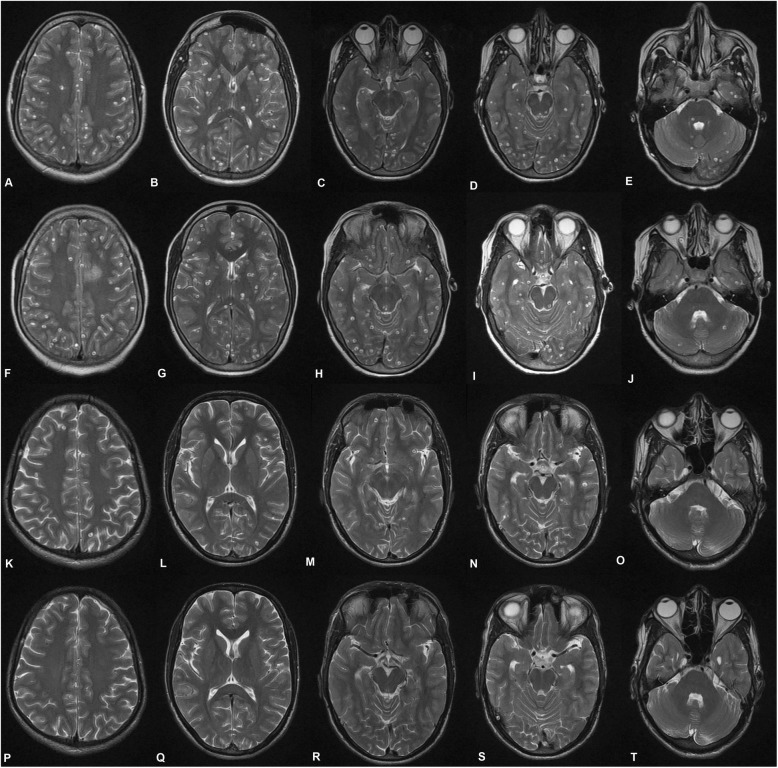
Axial T2W MRI images from a patient of disseminated cysticercosis. a-e Baseline images at different levels. f-j First follow-up at the same levels. k-o Second follow-up at the same levels. p-t Third follow-up at the same levels. A graded reduction can be noted from baseline to third follow-up. a, f, k, and p Centrum semiovale level. b, g, l, and q Basal ganglia level. c, h, m, and r First mesencephalic cut. d, i, n, and s Second mesencephalic cut. e, j, o, and t Middle cerebellar peduncle level
